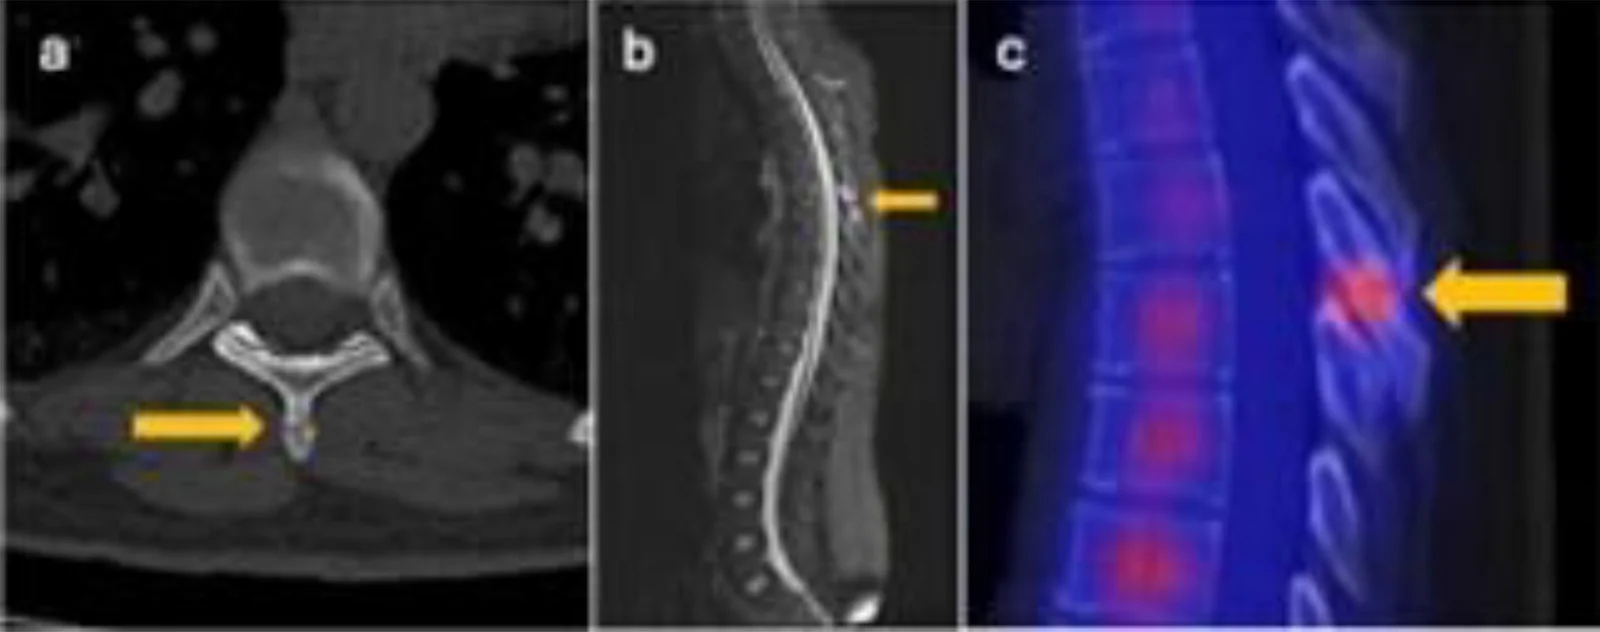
(a) CT, (b) short tau inversion recovery (STIR) sequence MRI and (c) single-photon emission computed tomography (SPECT) showing T6 spinous process non-union (defect indicated by yellow arrows) [60]; reproduced from Dietrich et al. [60], with permission
